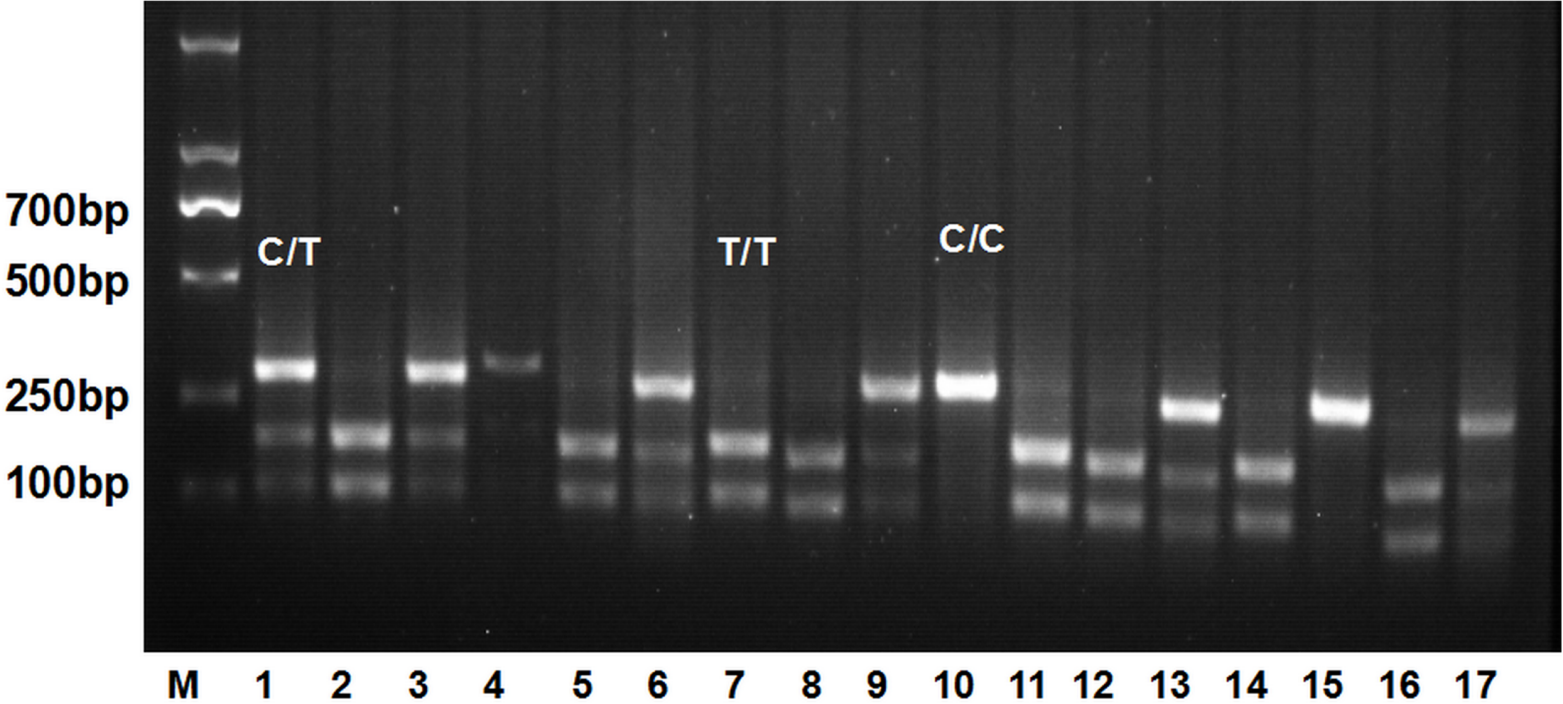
PCR-RFLP results of MTHFR C677T polymorphisms. Lane 1 is DNA marker. Lane 4, 10 and 15 are wild type genotype (CC). Lane 1, 3, 6, 9, 13, and 17 are heterozygous mutant genotype (CT). Lane 2, 5, 7, 8, 11, 12, 14 and 16 are homozygous mutant genotype (TT).
